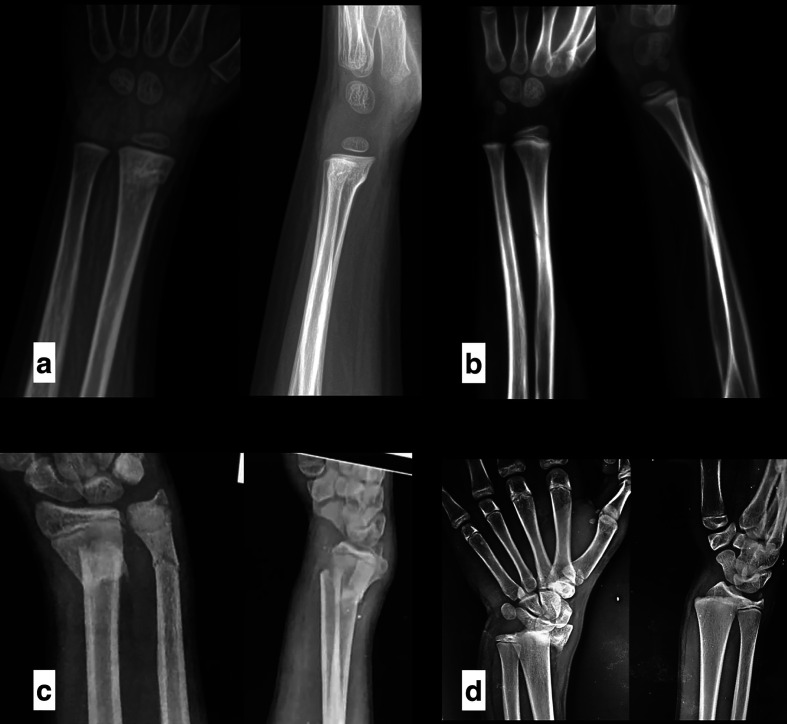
Representative radiographic patterns in pediatric distal radius fractures (AP and lateral views). (a) Torus (buckle) distal radius fracture (stable). (b) Minimally angulated distal metaphyseal fracture. (c) Completely displaced distal metaphyseal fracture. (d) Salter–Harris II distal radius fracture (physeal injury with metaphyseal fragment).
